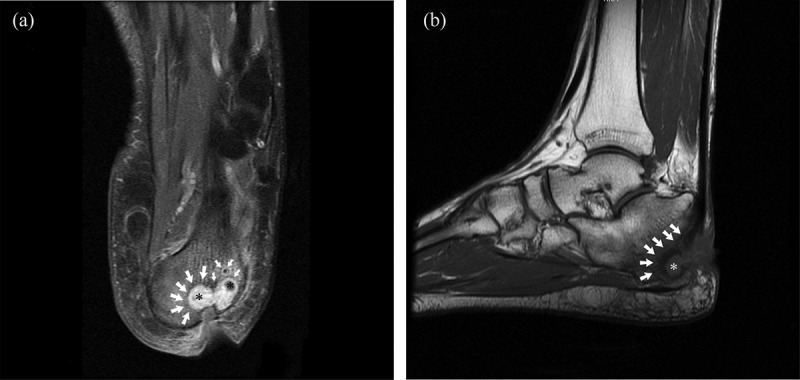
The planned resection level (white arrows) of the osteomyelitis on sagittal (a) and coronal (b) magnetic resonance imaging sections covering the abscess (asterisks). Actual resection can be predicted by including a few millimeters of bone covering the hypointense area over the nidus.
